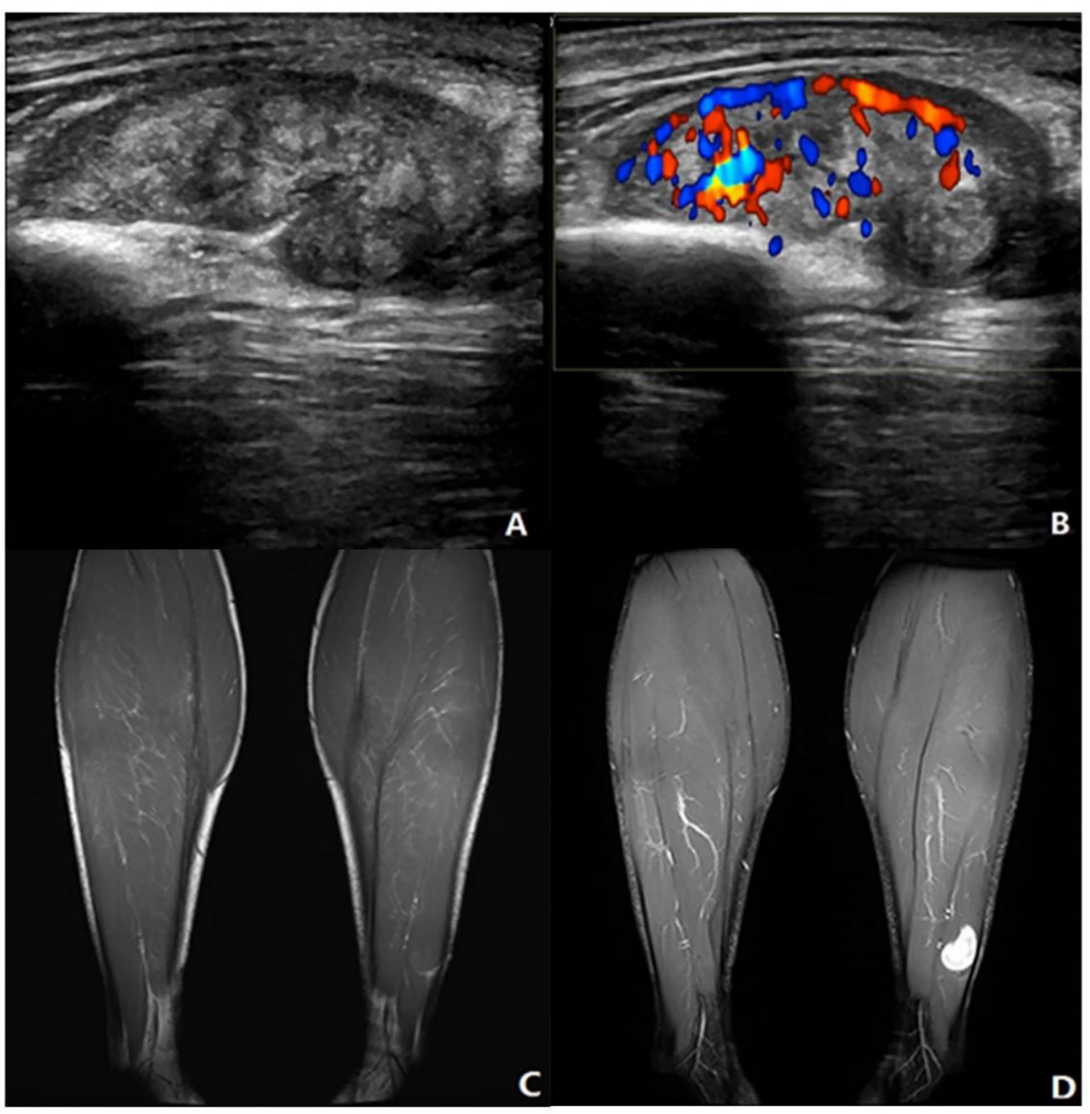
Neurinoma of lower extremity. A 41-year-old male presented with a mass in the left lower leg. (A) Longitudinal grayscale US showed a well-defined and heterogenous mass with maximum diameter of 34 mm in the peroneal brevis muscle. (B) Color Doppler imaging of the same area showed hypervascularity within the tumor, corresponding to type IV. (C) Coronal T1WI showed a homogeneous isointensity mass. (D) The lesion had homogeneous high SI on the coronal fat-suppressed T2WI with maximum diameter of 20 mm. (A, B) A score of 2 was assigned, indicating a benign tumor (true positive). (C, D) A score of 0 was assigned, indicating a benign tumor (true positive).
